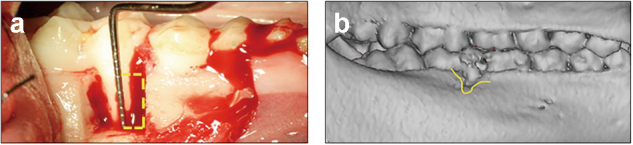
Establishment and evaluation of a minipig model of periodontitis. Generation of a minipig model of periodontitis. a We surgically created periodontal defects of 7 mm×5 mm×7 mm in the mesial region of mandibular first molars. b CT scans reveal obvious alveolar bone defects in the experimental region four weeks after the operation
